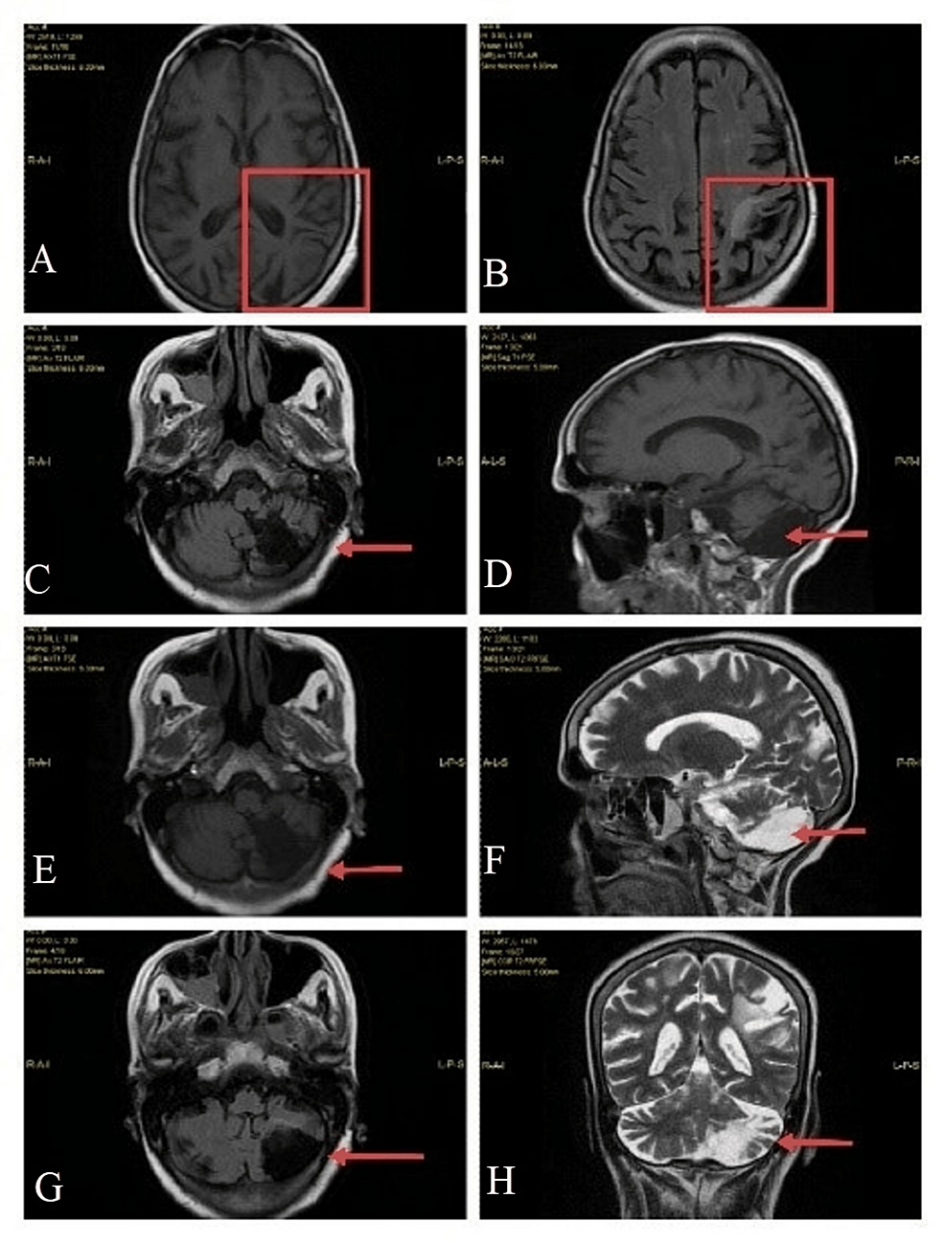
Postoperative MRI findings (A,B) gliotic changes in the left posterior parietal and occipital region; (C) gliotic changes (red arrow) in the left posterior parietal region; (D) encephalomalacia changes (red arrow) in the left cerebellar region; (E) gliotic changes (red arrow) in the left posterior parietal region; (F) encephalomalacia changes (red arrow) in the left cerebellar region; (H) encephalomalacia changes (red arrow) in the left cerebellar region; (G) gliotic changes (red arrow) in the left occipital region
